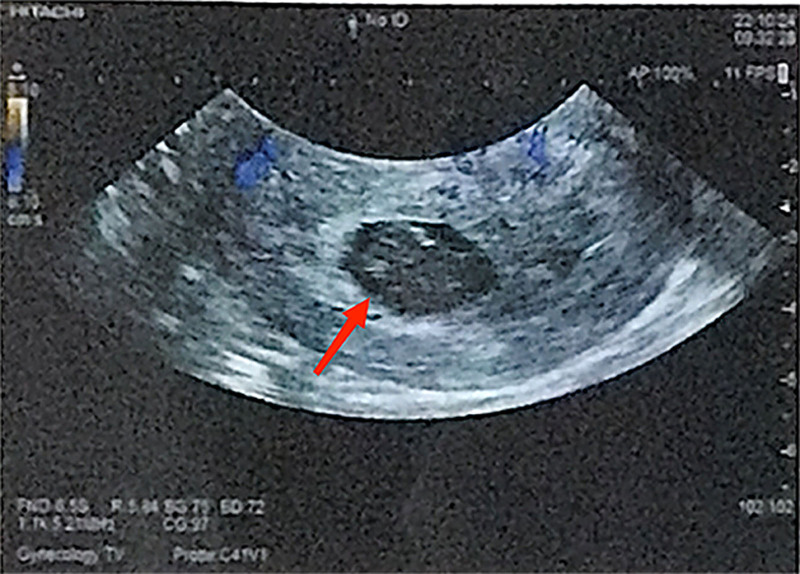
(October 2022) Transvaginal ultrasound showed an arrested fetus.
